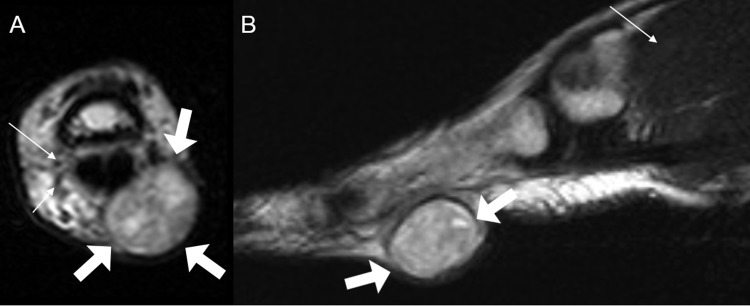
A 31-year-old man with digital schwannoma on the volar side of the 2^nd^ proximal phalanx of the hand. (A) On axial T2-weighted image, the mass shows multiple, small, low signal-intensity foci in a background of hyperintensity, representing the fascicular sign (thick arrows). The mass involves the digital neurovascular bundle. A normal palmar digital artery (long thin arrow) and normal palmar digital nerve (short thin arrow) are shown for comparison. (B) On sagittal T2-weighted image, the mass shows fascicular sign (thick arrows) in a background of hyperintensity compared to the skeletal muscle (thin arrow).
